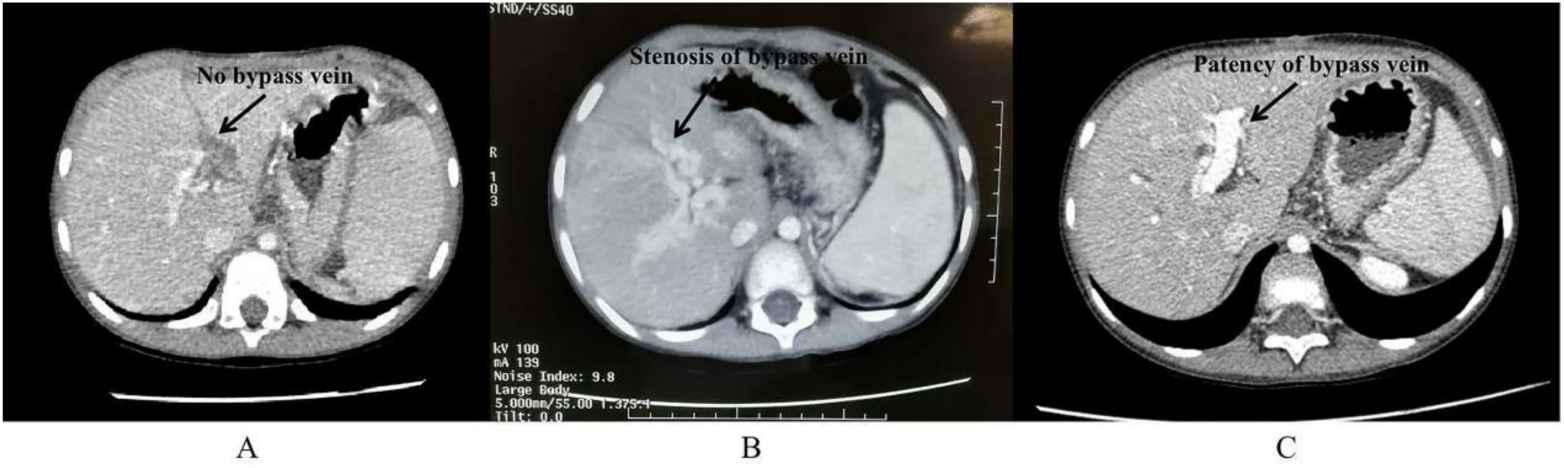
The CT after Rex shunt. The disappearance of the bypass vein is shown by CT (A); the stenosis of the bypass vein is shown by CT (B); the patency of the bypass vein is shown by CT (C).
